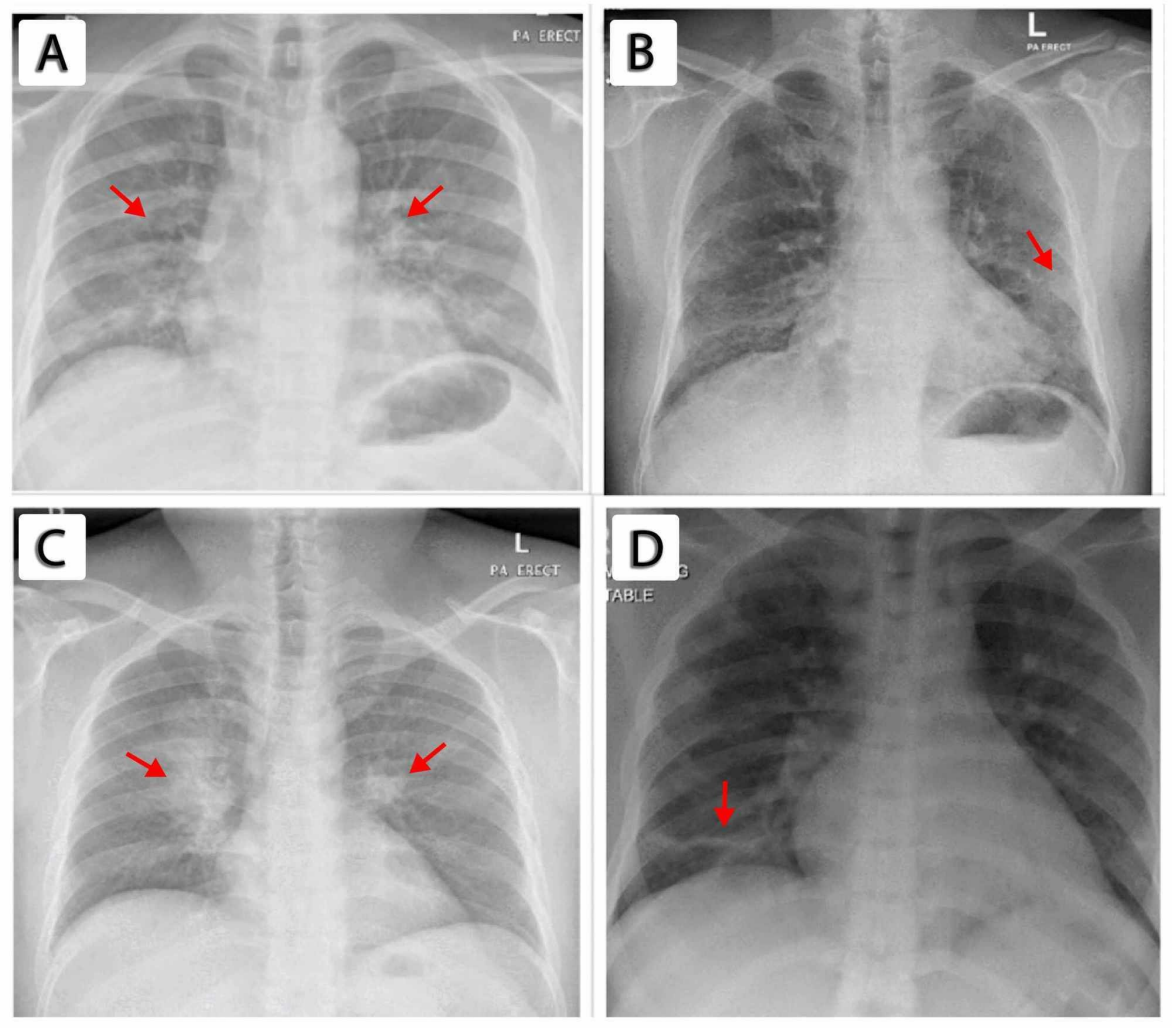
Chest X-rays of patients. (A) Patient 1 exhibiting bilateral patchy infiltrates and nonhomogenous opacities located mostly in the lower zones (red arrows). (B) Patient 2 exhibiting bilateral patchy infiltrates and nonhomogenous opacities located in the peripheral areas (red arrows). (C) Patient 3 exhibiting bilateral patchy infiltrates and nonhomogenous opacities more prominent in the middle zones (red arrows). (D) Patient 4 exhibiting bilateral patchy infiltrates and nonhomogenous opacities located peripherally (red arrows).
